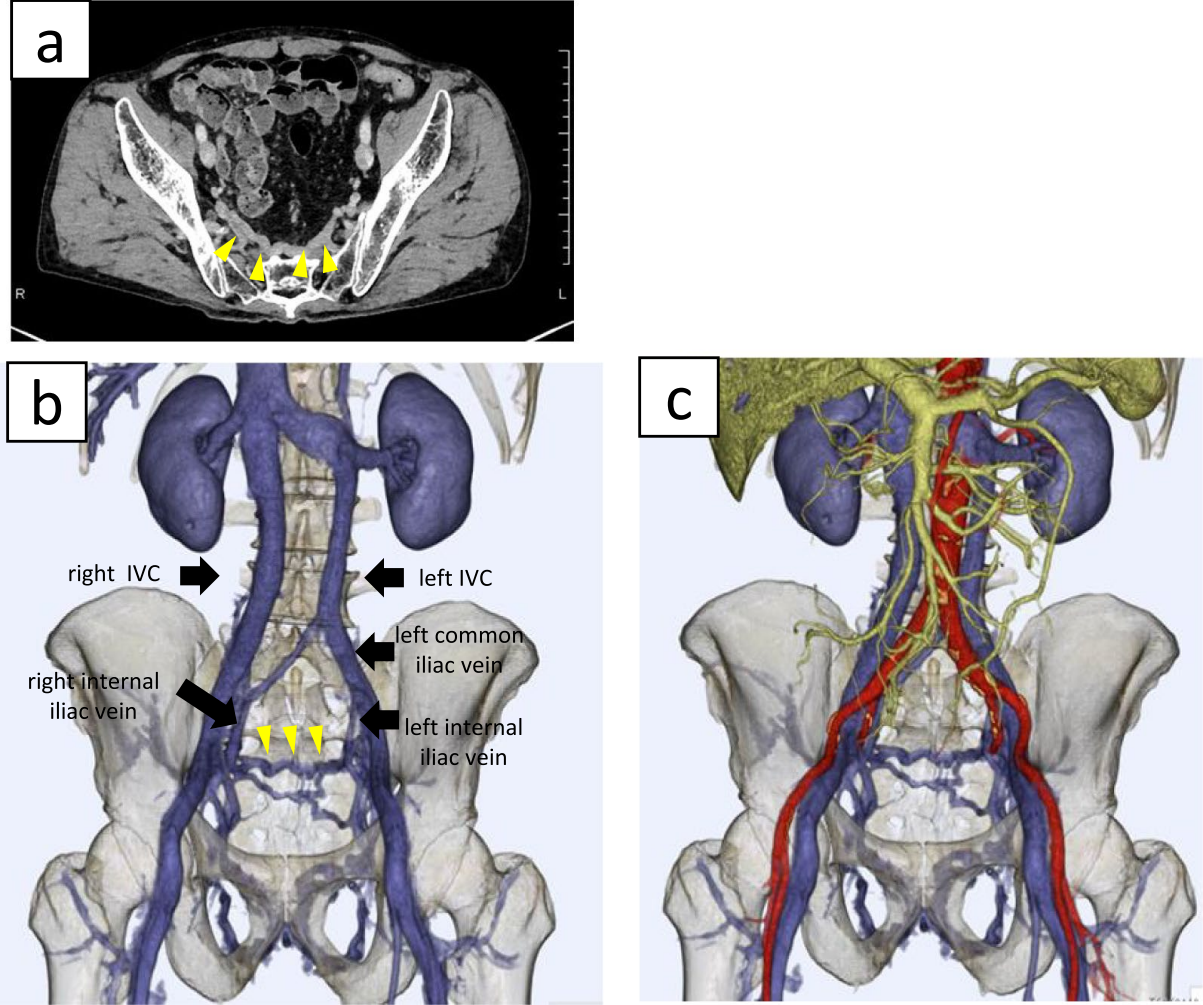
Pelvic computed tomography and three-dimensional computed tomography angiography. a, b A vein connecting the left and right internal iliac veins coursed anterior to the sacrum, forming an abnormal venous network (arrowheads). c 3D CT angiography of abdominal major vessels including portal vein, vena cava and abdominal aorta
